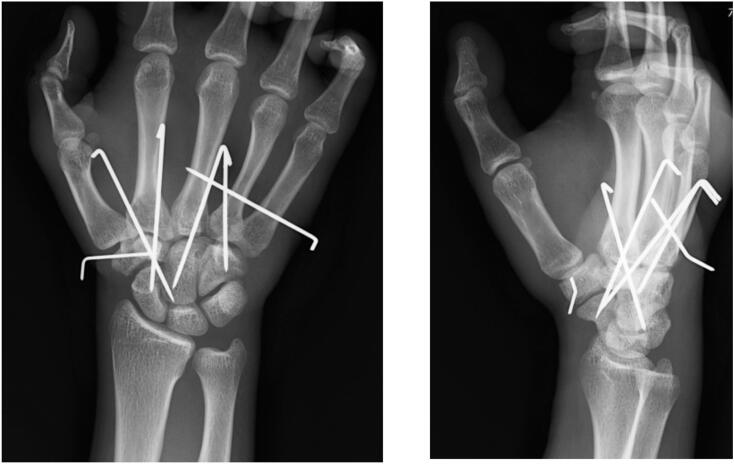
Postoperative X-rays after closed reduction and percutaneous pinning. The STT joint dislocation was reduced with longitudinal traction and transfixed with K-wires. CMC joints were stabilised with a K-wire from the fifth to the third metacarpal shafts.
